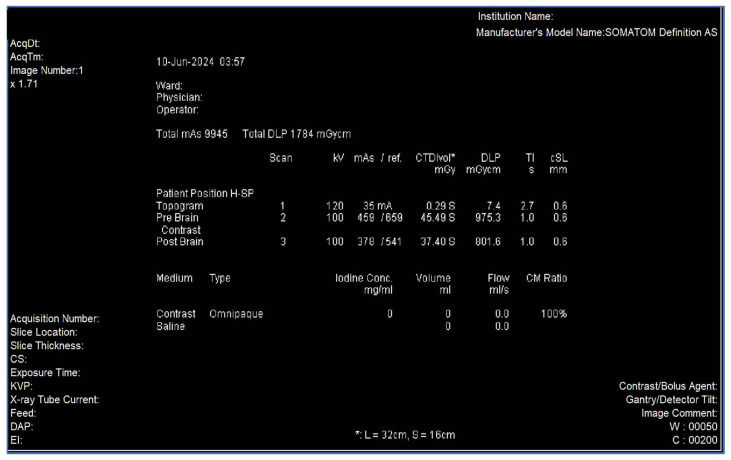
An example of a dose report for non-CE and CE CT brain.
